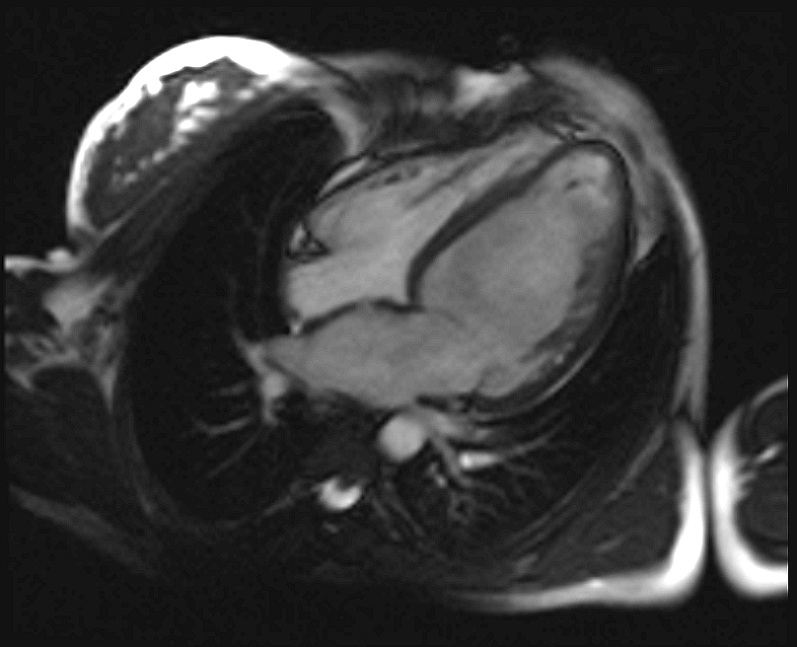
Visualization of trabeculae at MRI (sagital view)
